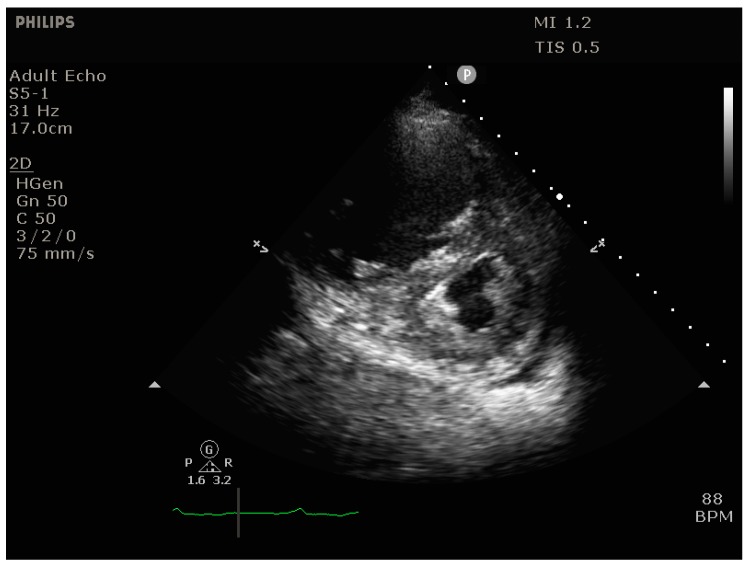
Transthoracic echocardiogram (parasternal short axis view) showing massive D-shaping of the interventricular septum.
